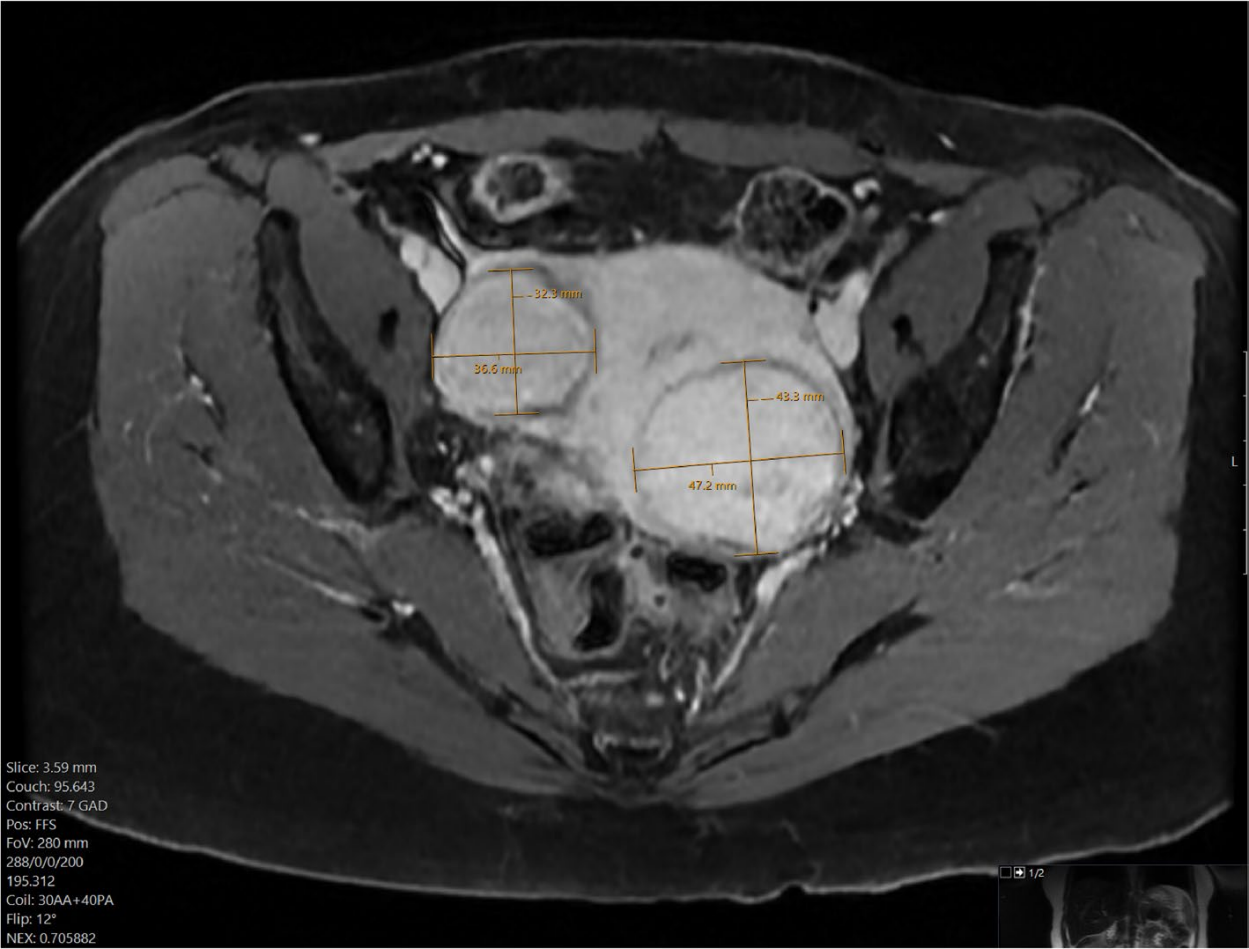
The patient’s MRI prior to embolization showed two uterine fibroids measuring 32.3 × 36.6 mm on the right and 47.2 × 43.3 mm on the left
